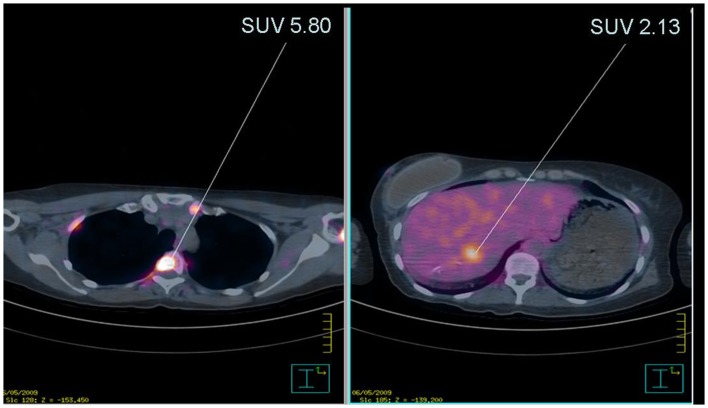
Anomalous concentration of ^18^F-FDG in index patient of family Y0029. Left panel: anomalous signal in bone projections of bone corresponding to predominantly intramedullary lesions. The arrow points to a lesion with SUV 5.80. Right panel: anomalous signal in the liver. The arrow points to a lesion with SUV 2.13.
